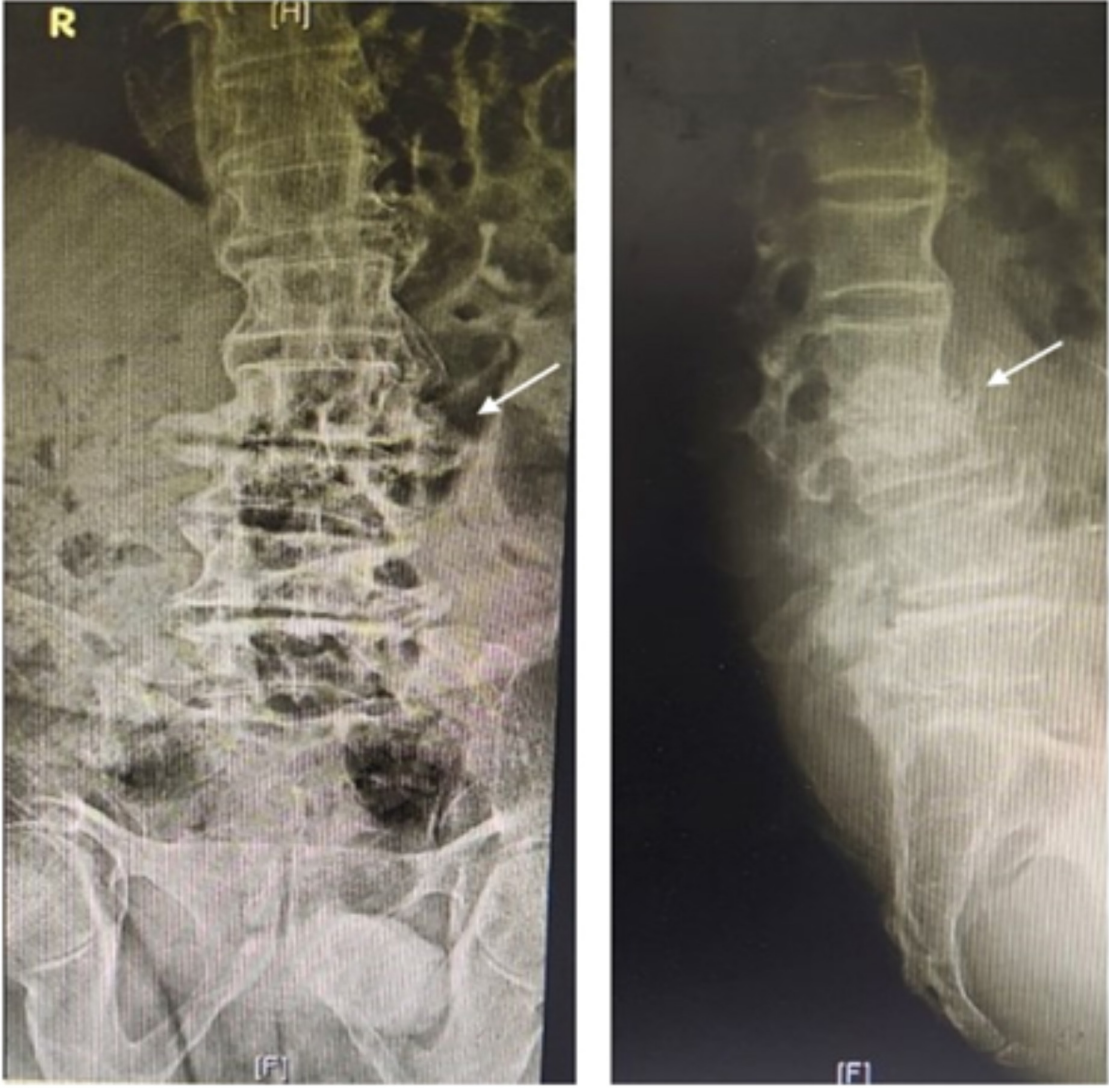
Lumbar spine radiographs (AP and lateral views) showing collapse of the L2–3 disc space with local kyphosis, apart from multilevel degenerative changes.
